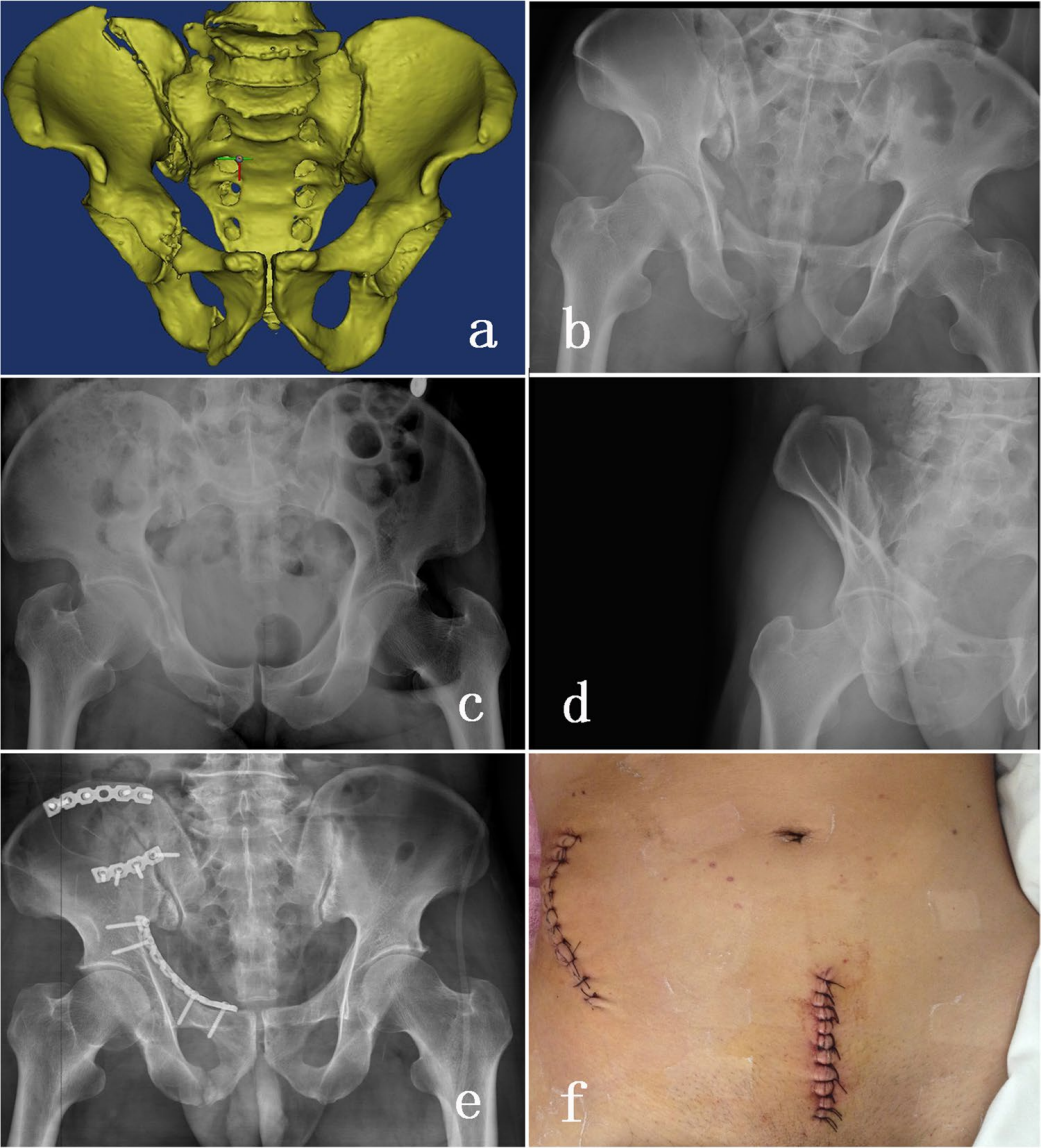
(a) Preoperative three-dimensional computed tomography reconstruction. (b) Pelvic AP view of an AO/OTA 61-C1 pelvic ring injury. (c,d) Pelvic inlet view and obturator oblique view showed the right pubic rami and the right iliac wing fracture. (e) The Stoppa approach combined with the iliac fossa approach were used to fix the fractures. (f) Postoperative imaging of the surgical wounds.
